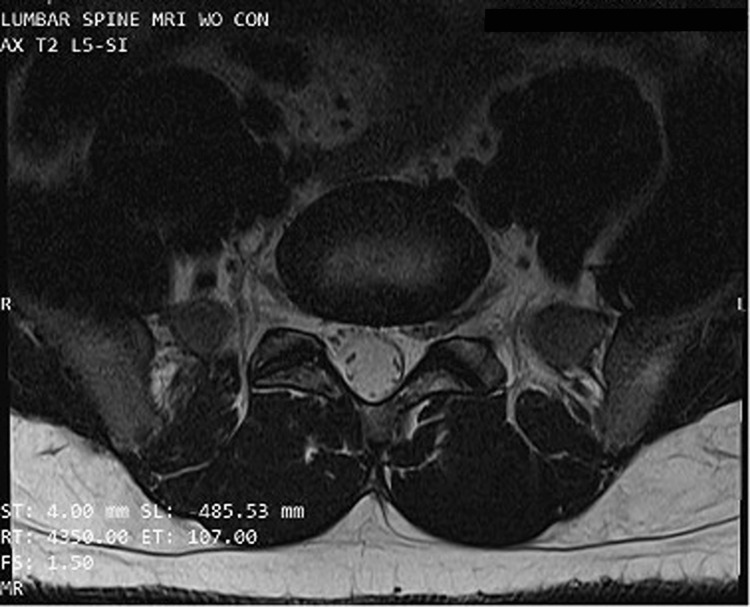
Axial magnetic resonance imaging view at the level of the fifth lumbar/first sacral (L5-S1) vertebrae reveals asymmetry of the left and right nerve root exit into the foramina.
